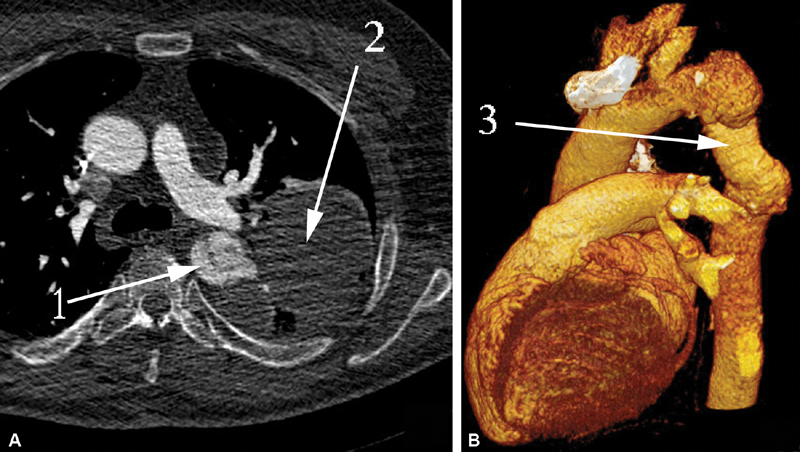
Computed tomography (CT) scan (
